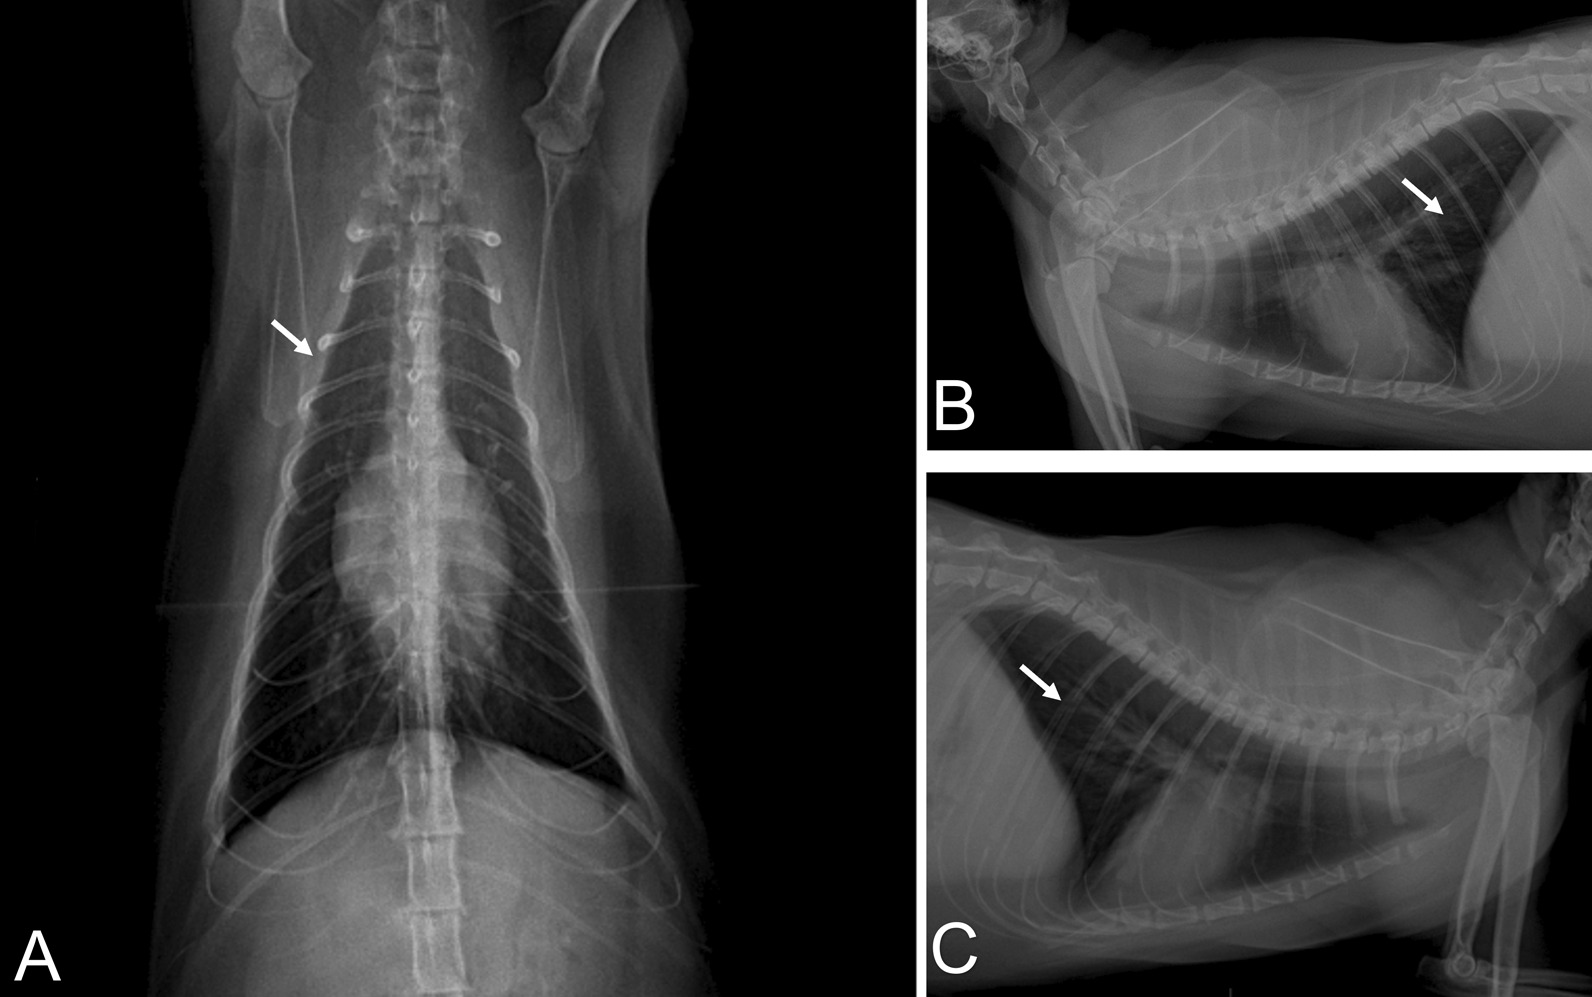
Radiographic views of ventrodorsal a lateral side (right and left, respectively), b, c increased radiopacity in the cranial and caudal lobes of both lungs accompanied by peribronchial infiltrate (white arrows) demonstrating incipient bronchitis
